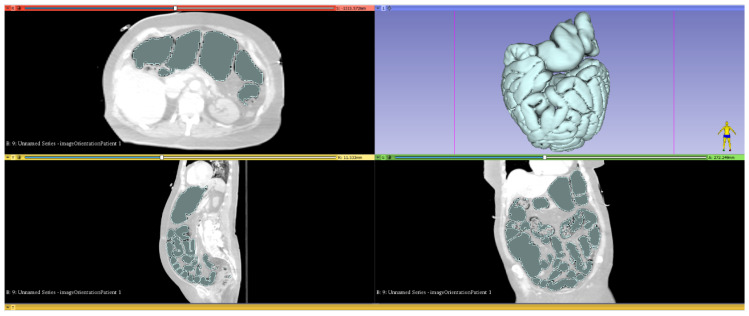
Visualization and segmentation of a CT scan utilizing analytic software.
